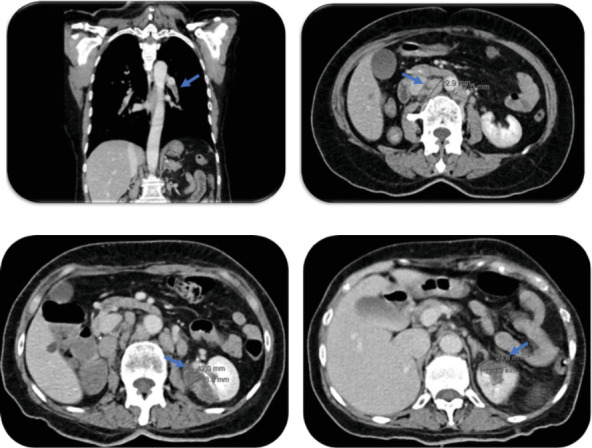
Contrast-enhanced computed tomography showing extensive bilateral pulmonary thromboembolism (a, blue arrow), retroperitoneal adenopathies, the largest located at the interaortocaval level with approximately 37 × 23 × 15 mm in diameter (b, blue arrow), areas of left kidney ischemia (c, blue arrow) and spleen (d, blue arrow).
